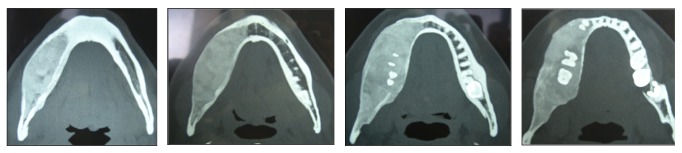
Axial sections by cone-beam computed tomography showing increase in the size of the mandibular body on the right side.
